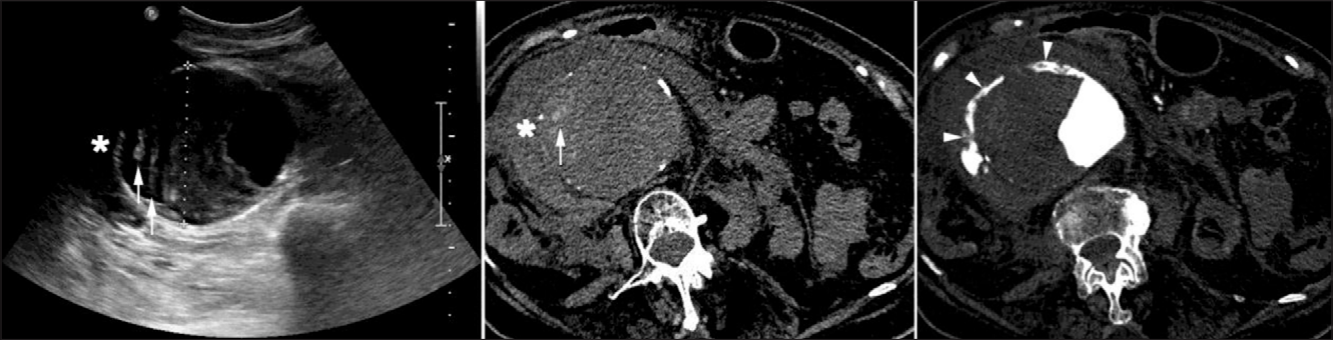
Transverse ultrasound (left panel) in an 89-year-old female admitted with shock showed a 11 cm AAA with a heterogeneous ILT, exhibiting an external hypoechoic crescent containing thick hyperechoic lines (arrows). Another hypoechoic structure surrounds the aorta (asterisk), suggesting a retroperitoneal hematoma. These intra-thrombus and retroperitoneal hematomas are confirmed by high attenuation (50–70 Hounsfield units) on unenhanced CT (centre panel). Contrast-enhanced CT (right panel) shows luminal contrast leaking into the periaortic hematoma through a channel dissecting the external ILT (arrowheads).
